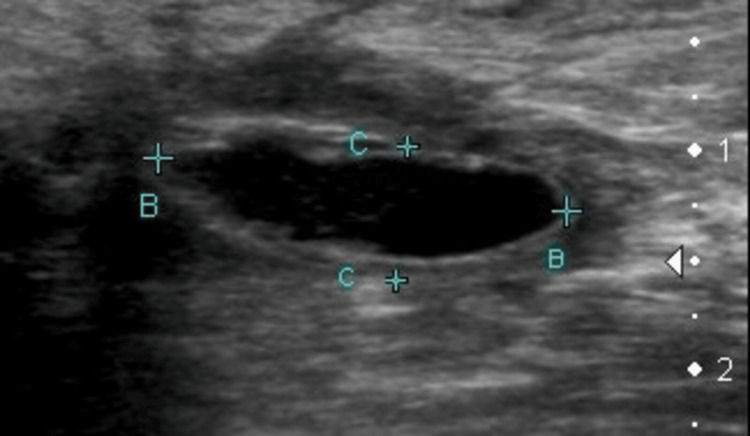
Ultrasound depiction of an intramuscular seroma showcasing its initial anechoic presentation and the potential evolution to exhibit thickened nodular margins. Case courtesy of Garth Kruger, Radiopaedia.org, rID: 18781 [8]. This image is used under the Creative Commons Attribution-NonCommercial-ShareAlike 3.0 Unported License. This work is licensed under a Creative Commons BY-NC-SA 3.0 License.
